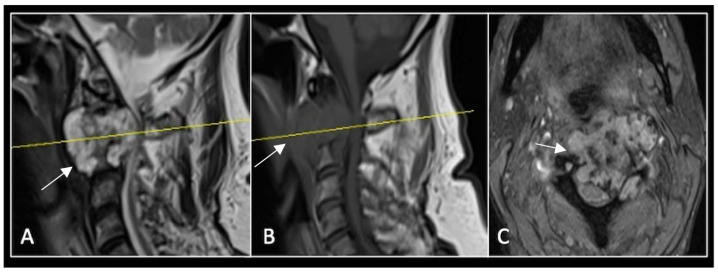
MRI demonstrating a destructive lobular lesion localised to the C2 vertebra (chordoma) (arrow). There is significant left-sided extension into the paravertebral tissues laterally and epidural space centrally with indentation of the cord. The yellow line on the sagittal images denotes the level at which the corresponding axial section was obtained. (A) T2-W sagittal; (B) T1W sagittal; (C) T2W axial.
